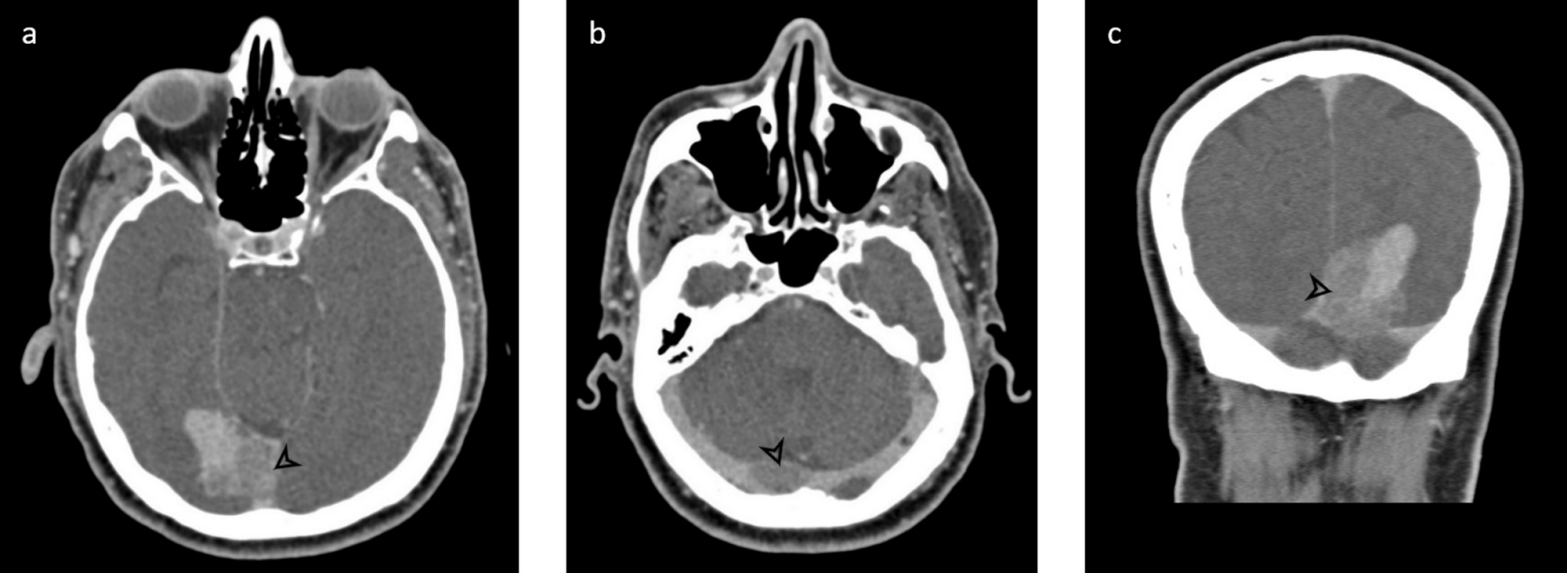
CT venogram Post-contrast CT venogram images along the middle (a) and inferior (b) portion of the mass, as well as coronal reconstruction (c). Tumoral invasion (arrowhead) is seen within the straight sinus, most posterior aspect of the superior sagittal sinus, torcula, and proximal right transverse sinus. The remaining portions of the transverse sinuses appear well opacified CT: computed tomography
